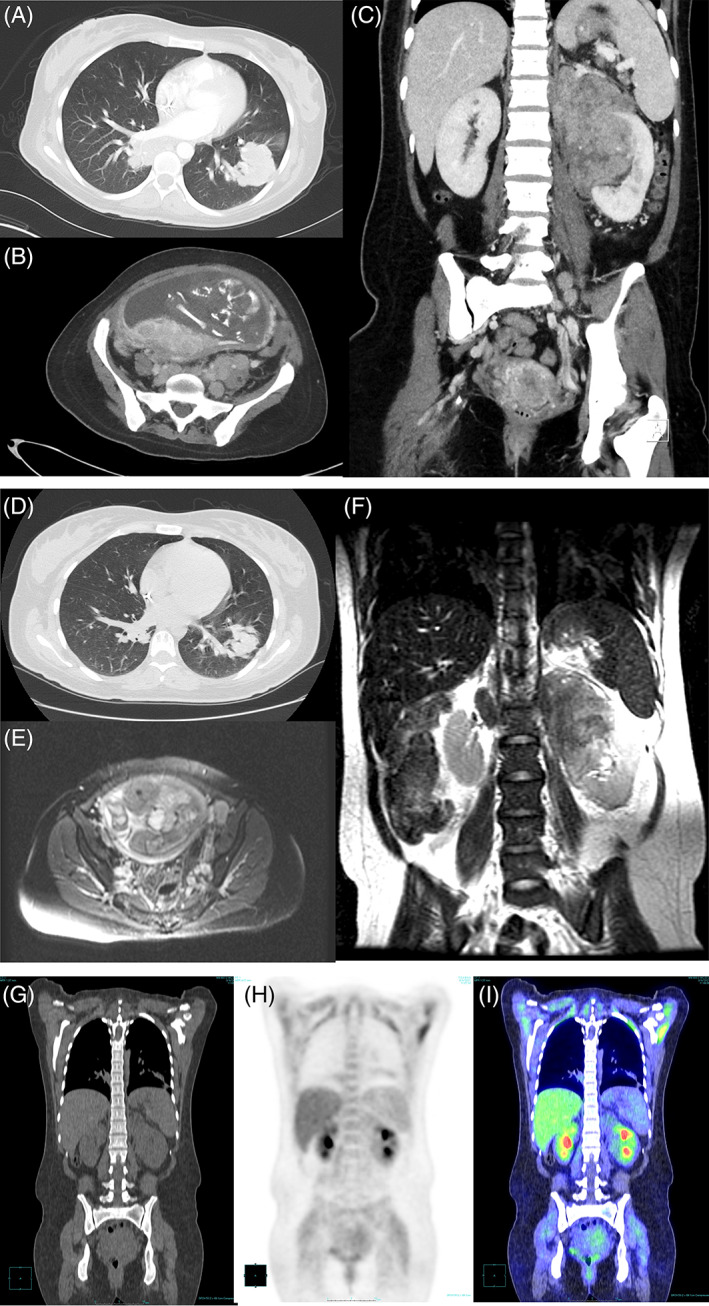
Imaging. (A) CT at 25 weeks gestation of right lower lobe mass measuring 4.5 × 2.4 cm and left lower lobe mass measuring 4.3 × 3.2 cm; (B) CT at 25 weeks gestation with axial view of extensive retroperitoneal and pelvic lymphadenopathy, and gravid uterus; (C) CT at 25 weeks gestation of conglomerate of para‐aortic lymphadenopathy in the upper abdomen with necrotic features measuring approximately 11 × 4.4 cm; (D) CT chest at 32 weeks gestation of right infrahilar location smaller measuring 2.3 × 3.2 cm, and left lower lobe parenchymal mass measured 3.6 × 3.1 cm; (E) MRI abdomen at 32 weeks gestation of axial view of smaller retroperitoneal mass; (F) MRI abdomen at 32 weeks gestation of left sided para‐aortic lymphadenopathy smaller measuring 8.3 × 6.0 × 5.3 cm; (G) PETCT coronal view at 2 weeks postnatal of right lower lobe perihilar opacity measuring 2.2 cm; redemonstration of left renal hilar mass; (H) Coronal re‐formatted PET/CT image at 2 weeks postnatal; and (I) PET/CT at 2 weeks postnatal of fused coronal view with decrease in size of left renal hilar mass at 4.1 cm with low‐level SUV max 1.5. CT, Computerized tomography; MRI, magnetic resonance imaging; PET, positron emission tomography [Color figure can be viewed at wileyonlinelibrary.com]
